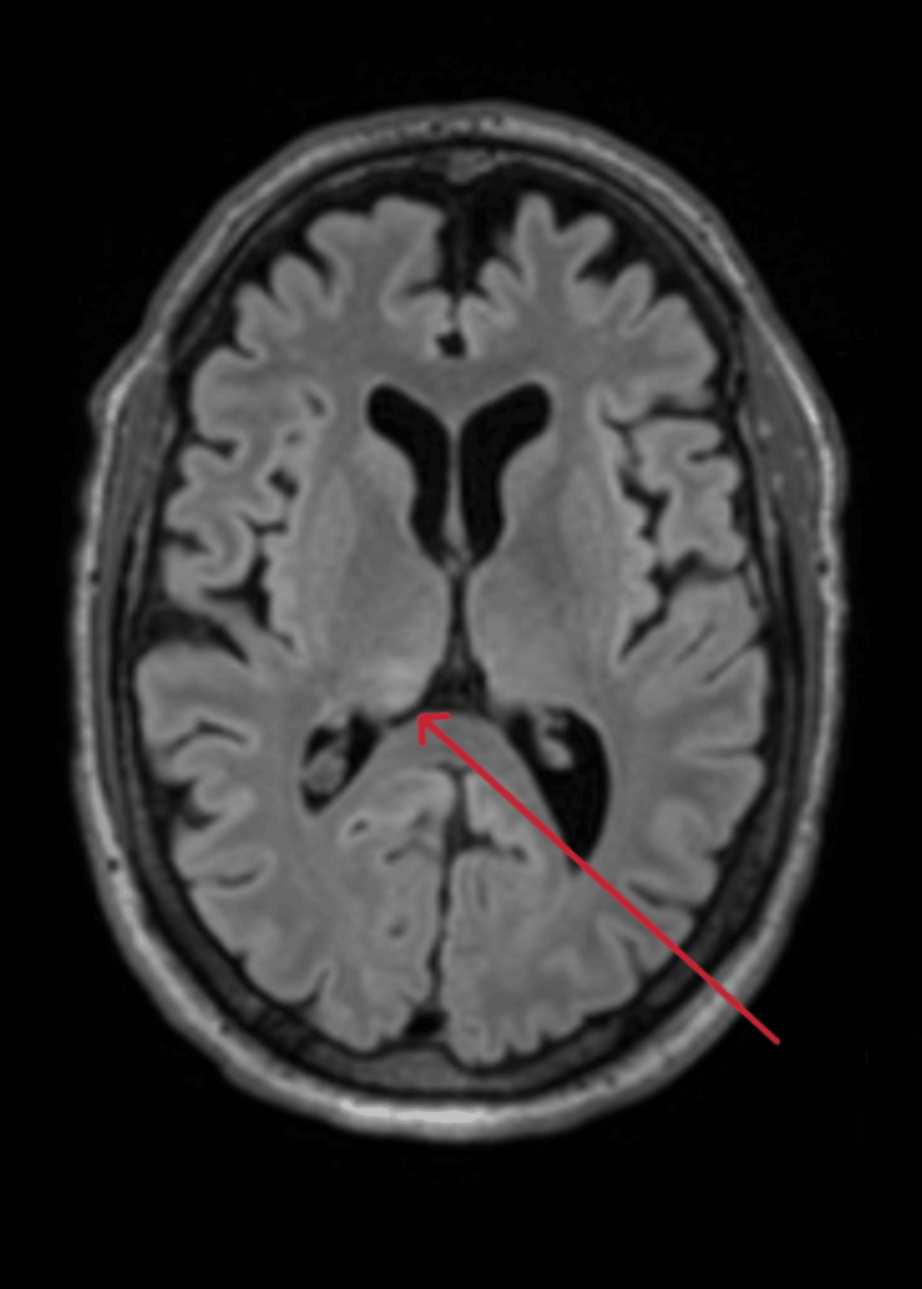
Axial brain MRI showing increased FLAIR signal in the right thalamus MRI: Magnetic resonance imaging; FLAIR: fluid-attenuated inversion recovery
